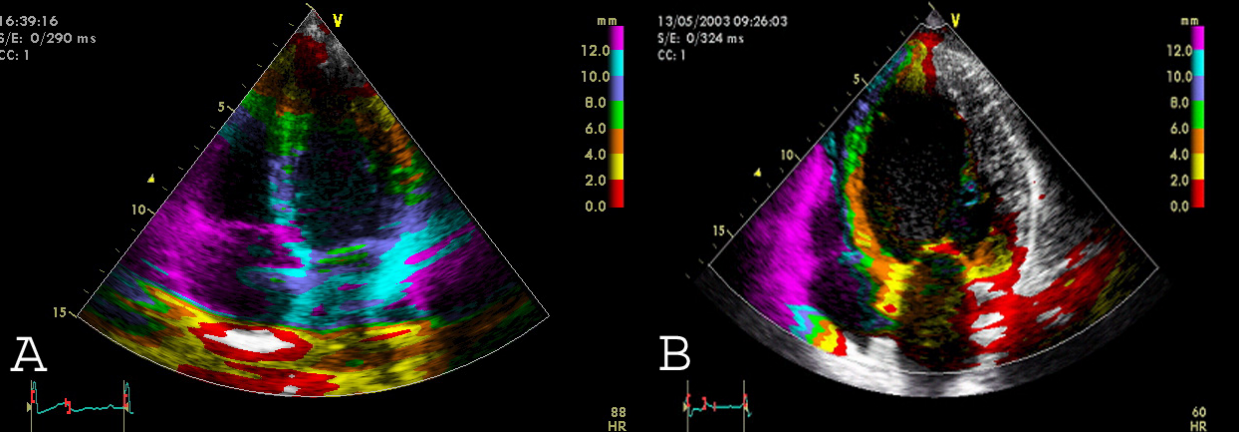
Tissue Tracking allows the visualization of longitudinal motion in each myocardial segment. Images are from the apical four chamber view. A) Normal control patient. There are normal colour-coded displacement values in the lateral and septal segments, with physiologically higher values in the more basal segments and lower values towards the apex. B) Tissue Tracking in a patient with dilated cardiomyopathy. There is a dilated left ventricle with "baseball shape" and reduced displacement values and no basal-apical gradient (max displacement = 8 mm) in the septal segments and DLC in the lateral wall (no colour-coding) indicating asynchrony of the lateral wall.
